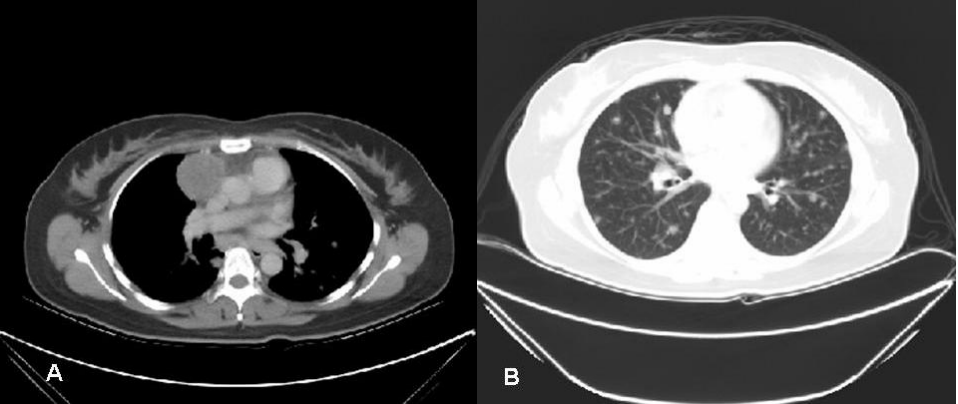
Chest CT scan. A. a right anterior mediastinal shadow. B. multiple pulmonary nodular lesions.
